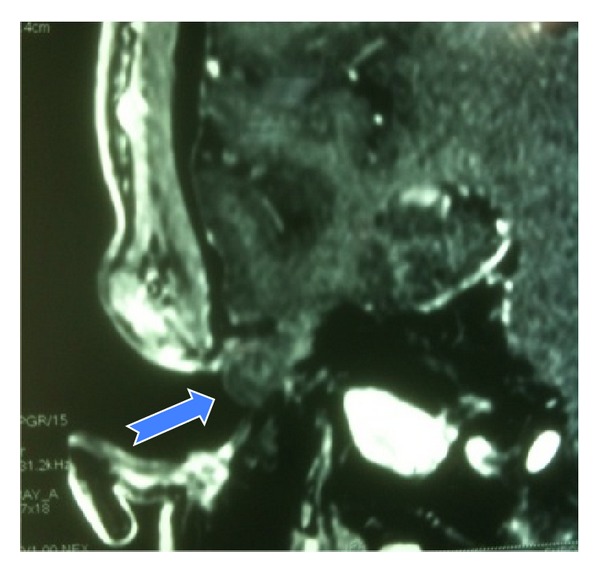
Preoperative imaging; T1 weighted contrast-enhanced MRI (coronal view) revealing the temporal bone encephalocele (blue arrow).
